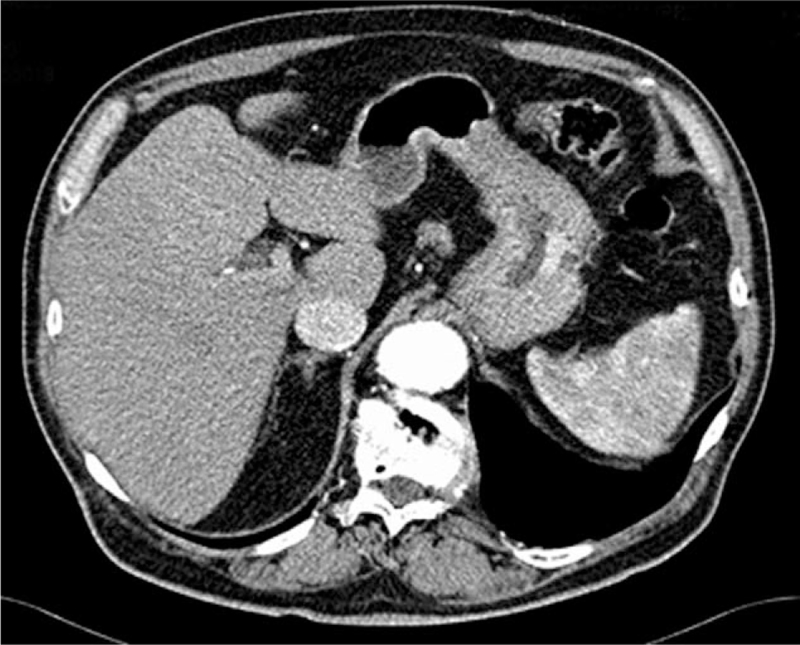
Computed tomography scan 5 months after surgery: gastric thickening at the greater curvature side of 1.5 cm in size.
